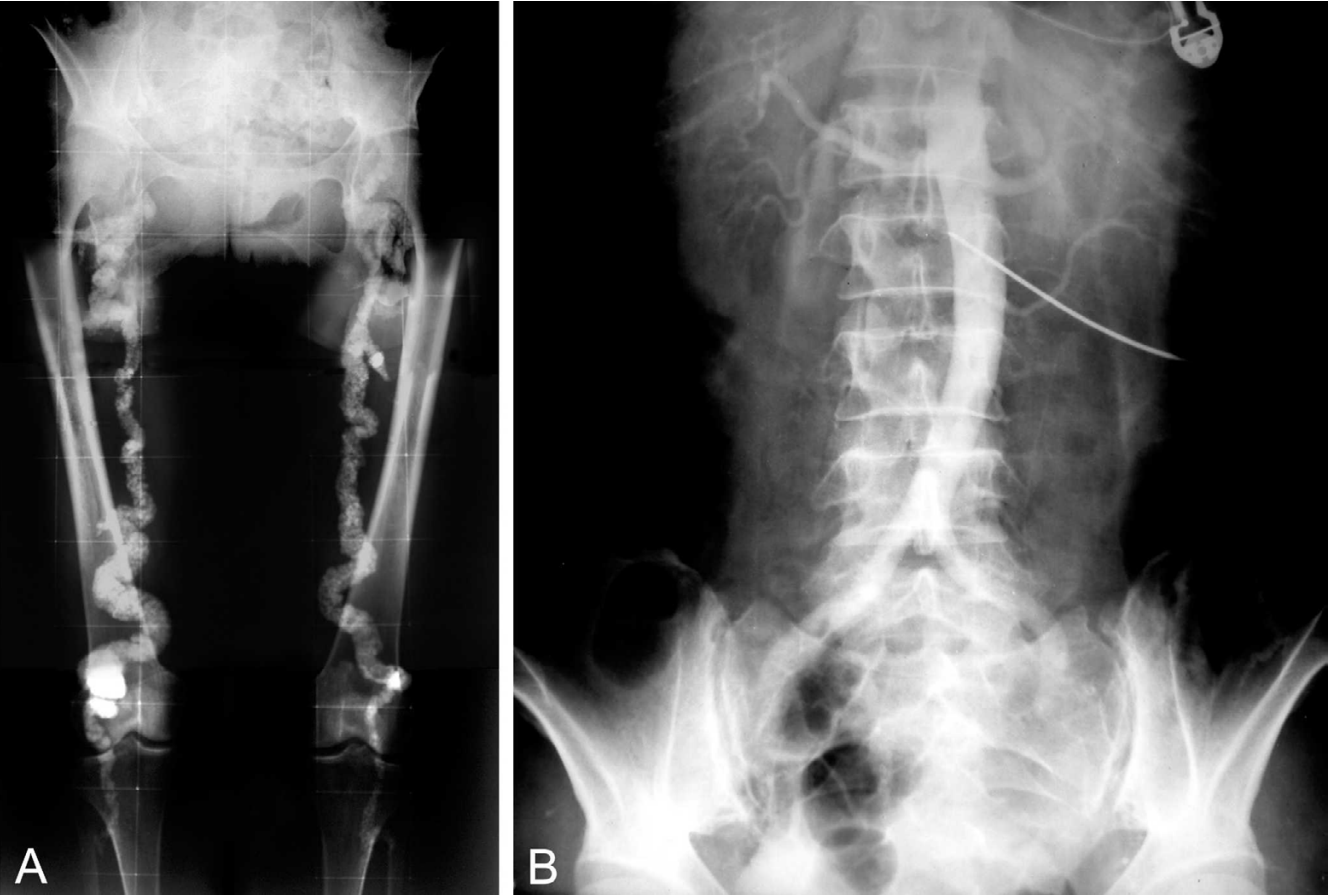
A. Pelvic and lower extremity radiograph shows extensive calcification of the femoral arteries. B. Translumbar aortography shows near-total obstruction of the femoral arteries.
